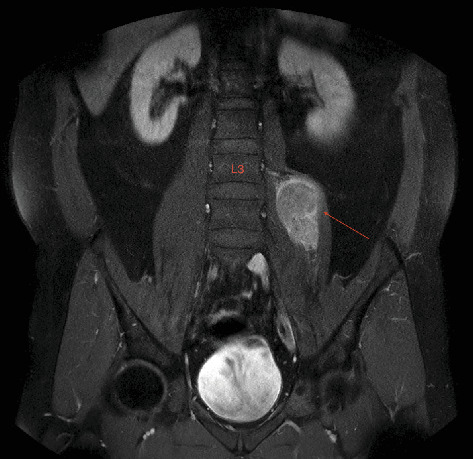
Mass lesion in the psoas muscle (red arrow) in which the upper border is seen as the third lumbar (L3) vertebra (coronal T1-weighted MRI).
